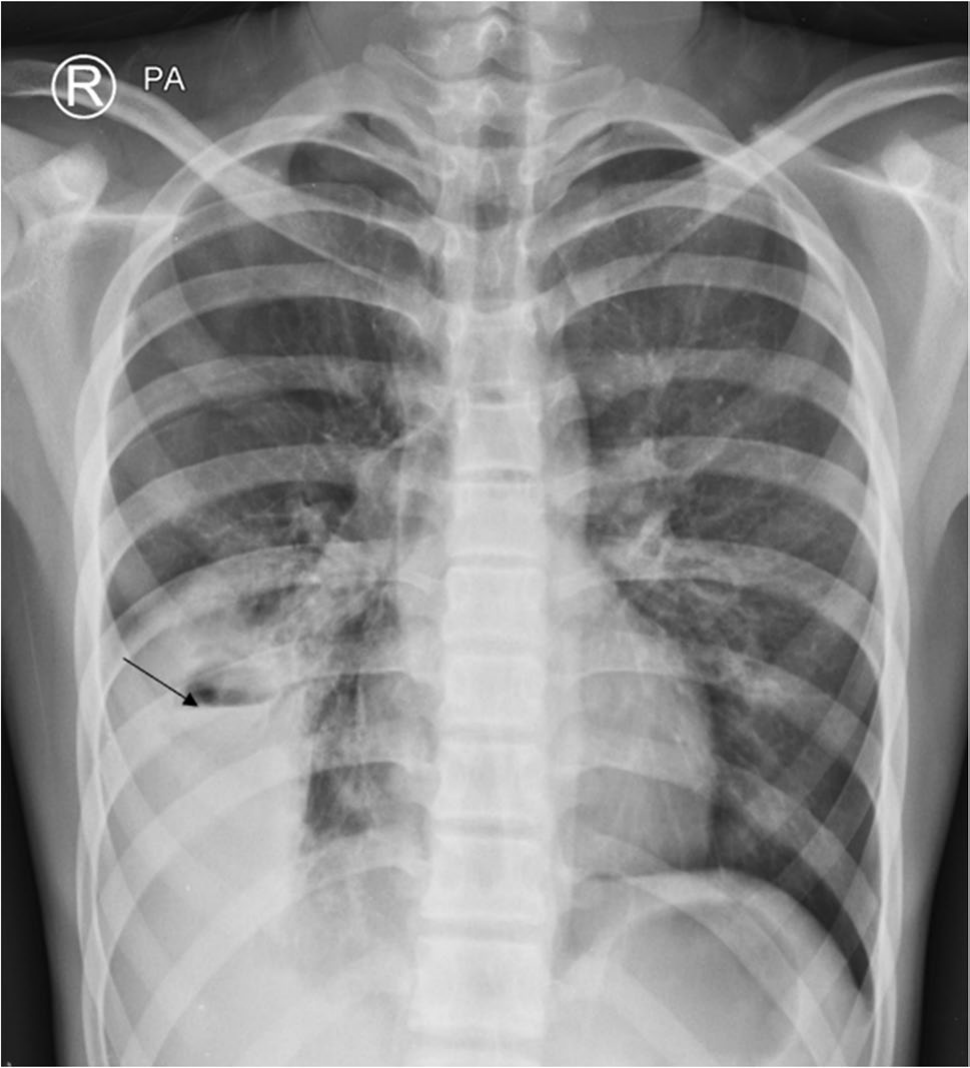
Posteroanterior chest radiograph in a 15-year-old boy with amoebiasis who presented to the emergency department complaining of breathlessness for the preceding 10 days shows opacity with an air-fluid level (*arrow*) in the right lower lobe (amoeboma) and a right-sided pleural effusion (figure from “A Rare Case of Primary Pulmonary Amoebiasis without Gastrointestinal Involvement: A Case Report” by Nugroho et al.; licensed under CC BY-SA; original cropped and annotated)
